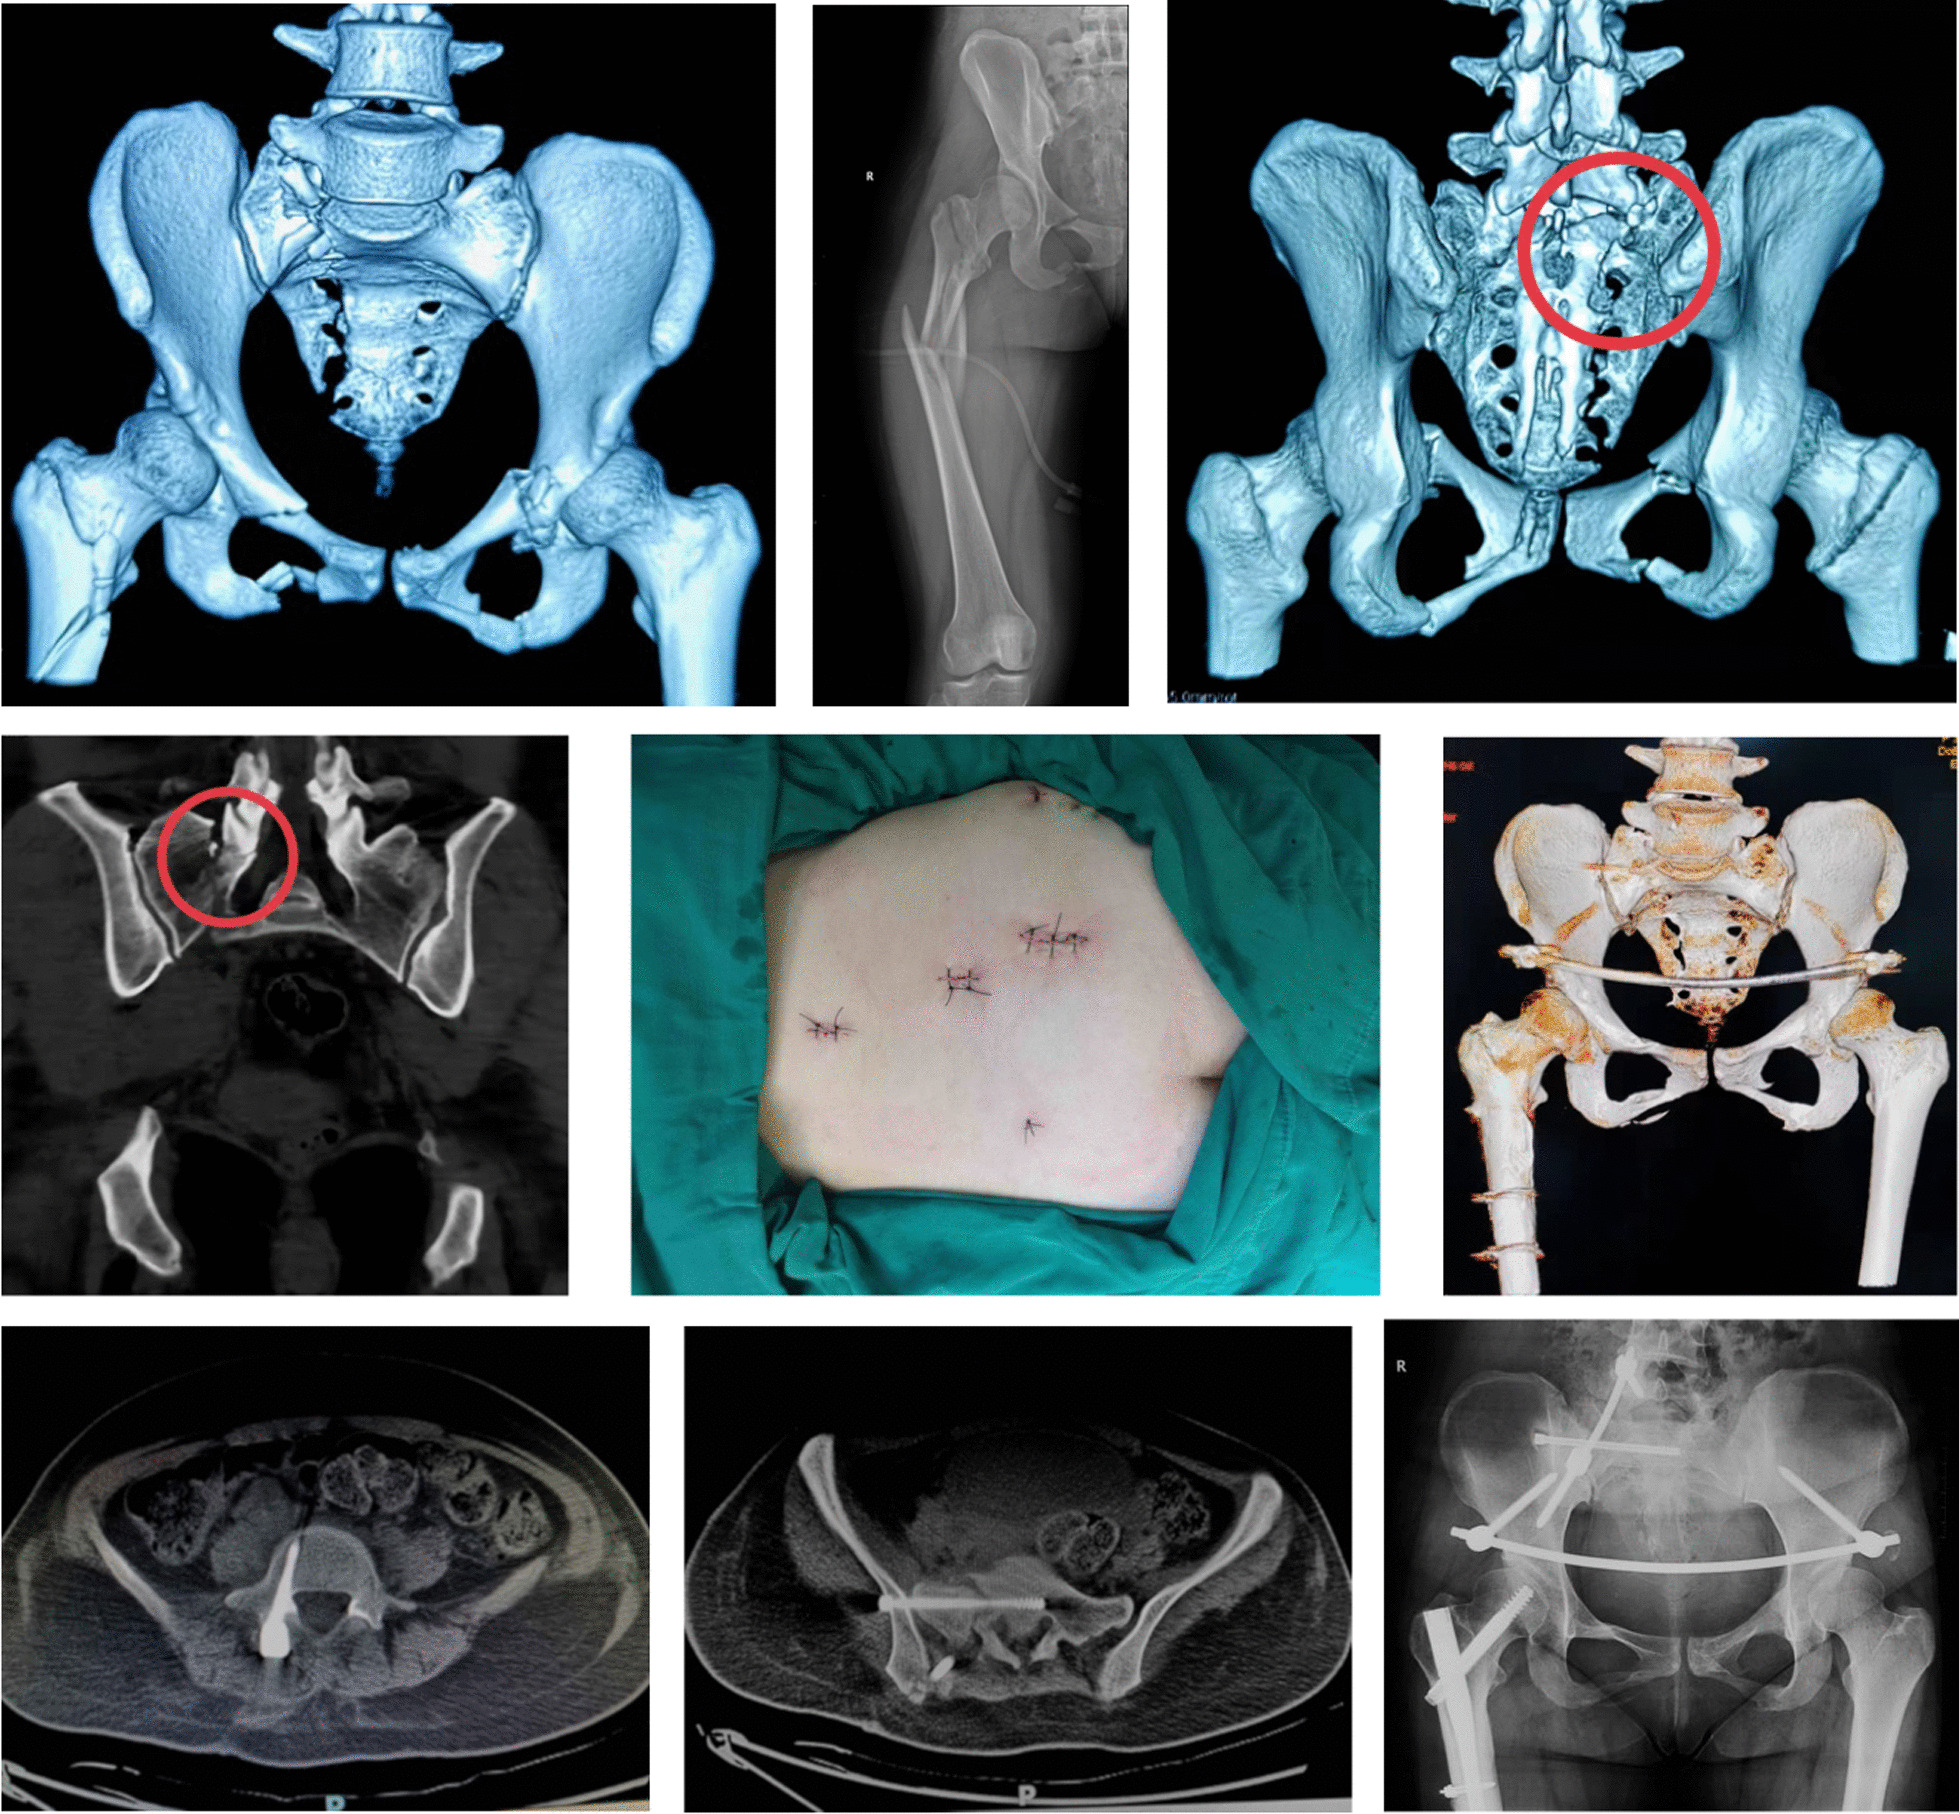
Female, 34-year-old, a fall from height. A The CT reconstruction view on admission showed a vertically displaced fracture on right sacrum associated with bilateral rami fractures (Tile type C1). B The radiograph showed a comminuted subtrochanteric fracture on right femur. C,D The areas in the red circles on CT views revealed a fracture on right S1 articular process (Isler type IIb). E The surgical incisions of posterior pelvic ring after minimally invasive TOS with TiRobot assistance. F Postoperative CT reconstruction image. The anterior pelvic ring was stabilized by INFIX and the satisfactory reduction of the pelvic fracture and the femoral fracture was achieved. G,H Axial CT view demonstrated the pedicle screw and the sacroiliac screw were both in the cancellous bone. I Postoperative 6-month radiograph showed fracture healing
